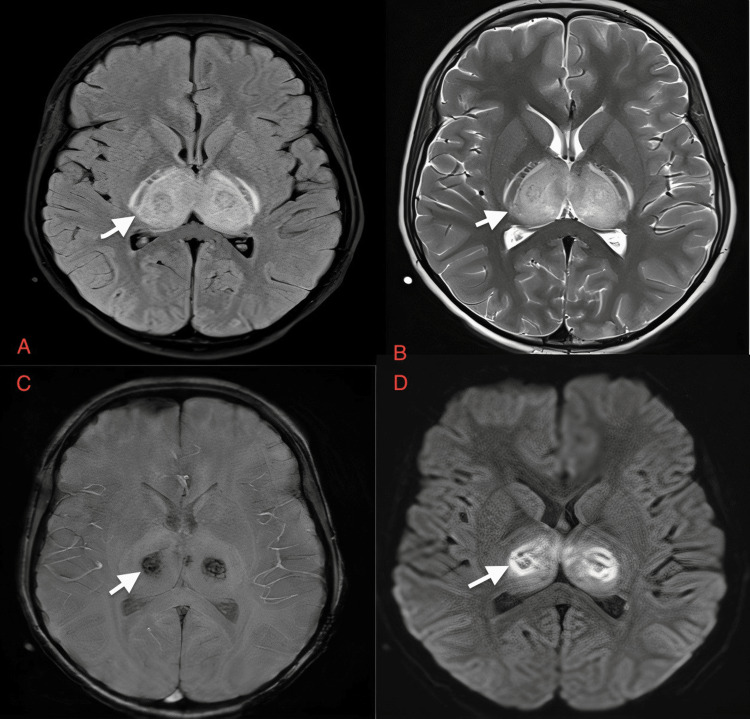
Case 8: Symmetrical hyperintensities are seen on FLAIR and T2W (white arrows in A and B, respectively) images in both thalami in a nine-year-old male patient on day one of presentation with dengue encephalitis. Central areas of blooming indicating hemorrhage are seen on SWI (C). Diffusion restriction is also seen on DWI (white arrow in D). FLAIR: fluid-attenuated inversion recovery sequence; T2W: T2-weighted image; SWI: susceptibility-weighted image; DWI: diffusion-weighted image
